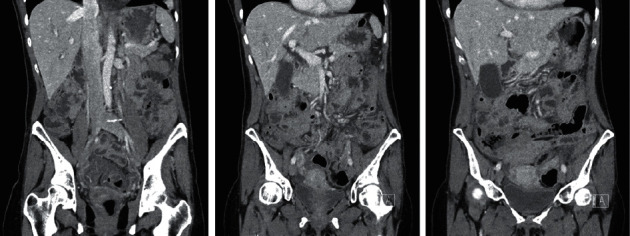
Computed tomography (CT) images demonstrated diffuse large bowel thickening consistent with ischemic colitis.
